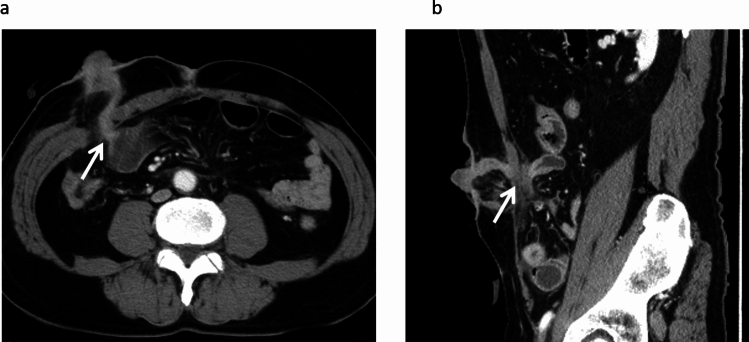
Representative computed tomographic image of stoma outlet obstruction. a Axial view showing outlet obstruction at the site where the stoma penetrates the abdominal wall. b Sagittal views showing outlet obstruction at the same site
